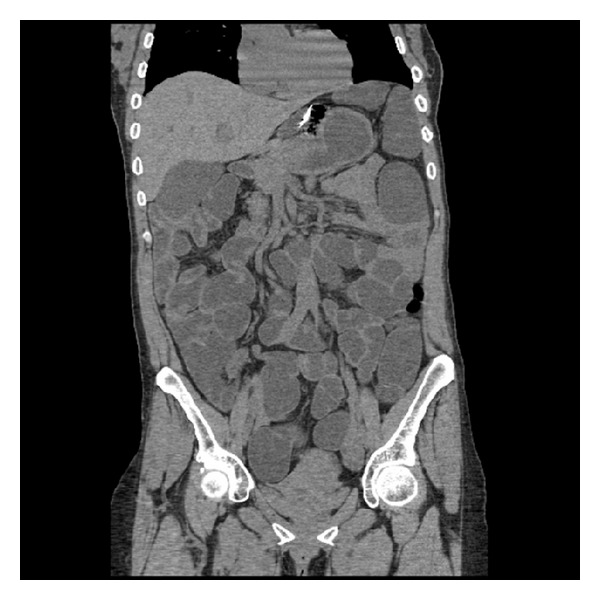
Computed tomography abdomen image showing nondilated bowel loops.
